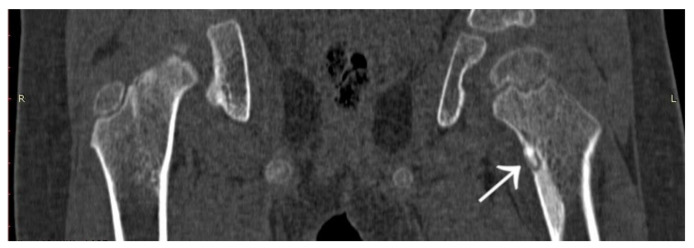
CT scan of the hip joints showing a focus of OO (arrow).
